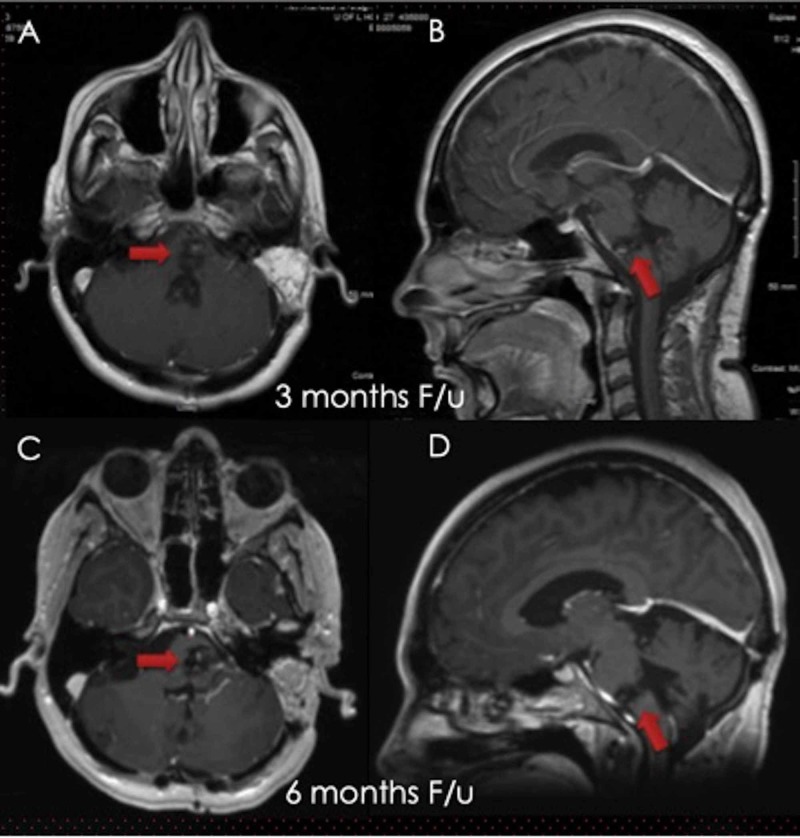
Follow-up MRI (T1W with contrast) at three months (A&B) and six months (C&D) showed a stable size of the resection cavity of the pontomedullary lesion with no recurrence or repeat hemorrhage (red arrows). MRI: magnetic resonance imaging
